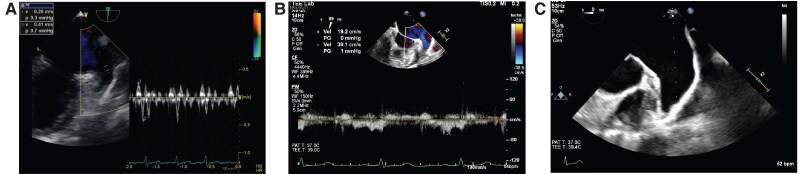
(*A*) Pre-operative transoesophageal echocardiography image at the first session. No thrombus inside the left atrial appendage was detected. Pulse Doppler showed that the average left atrial appendage flow velocity was 33.5 cm/s (emptying velocity 26.0 cm/s, filling velocity 41.0 cm/s). (*B*) Pulse Doppler image obtained by transoesophageal echocardiography at the fourth session. Average left atrial appendage flow velocity was decreased to compare with previous examination (average velocity 23.4 cm/s, emptying velocity 19.5 cm/s, and filling velocity 27.3 cm/s). (*C*) Transoesophageal echocardiography image of the left atrial appendage at the fourth session. No thrombus nor spontaneous echo contrast was detected.
